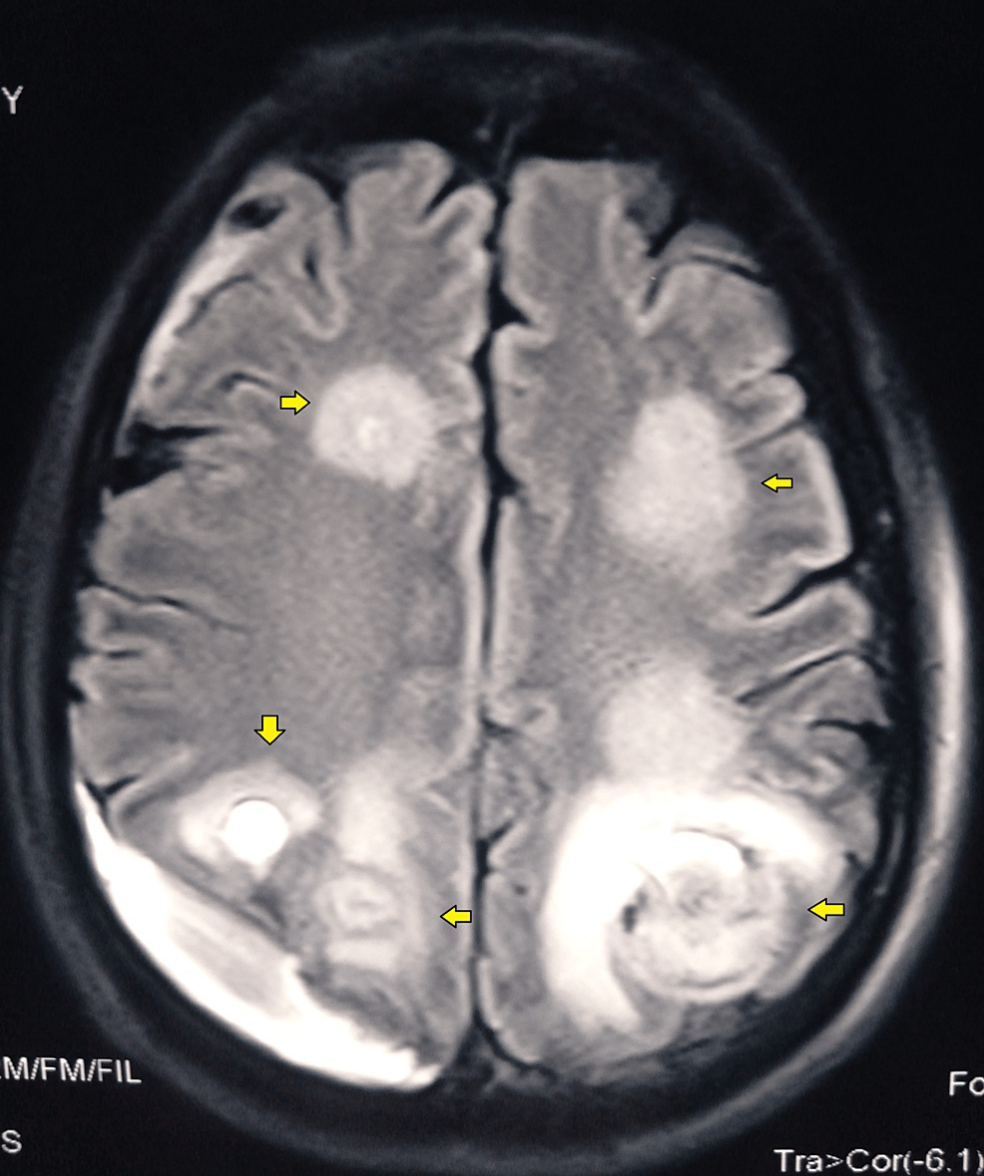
MRI brain ( FLAIR sequence) showing predominately hyperintense lesion involving fronto-parieto-temporo-occipital lobes (yellow arrow) FLAIR- fluid-attenuated inversion recovery
